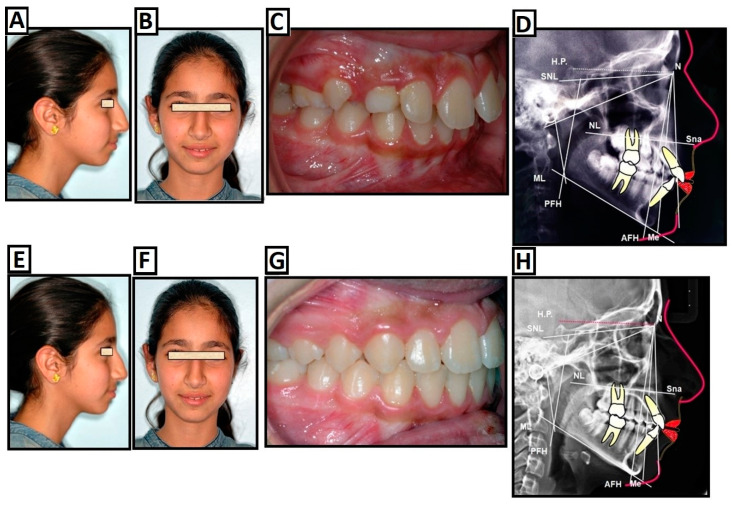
(A–F) Camouflage therapy of a class II dysgnathy, non-extraction, by destabilizing the teeth in the upper jaw. (A–D) Before treatment: (A) lateral photo of the patient, (B) frontal photo of the patient, (C) intraoral photo, and (D) cephalometric radiograph. (E–H) After treatment: (E) lateral photo of the patient, (F) frontal photo of the patient, (G) intraoral photo, and (H) cephalometric radiograph.
